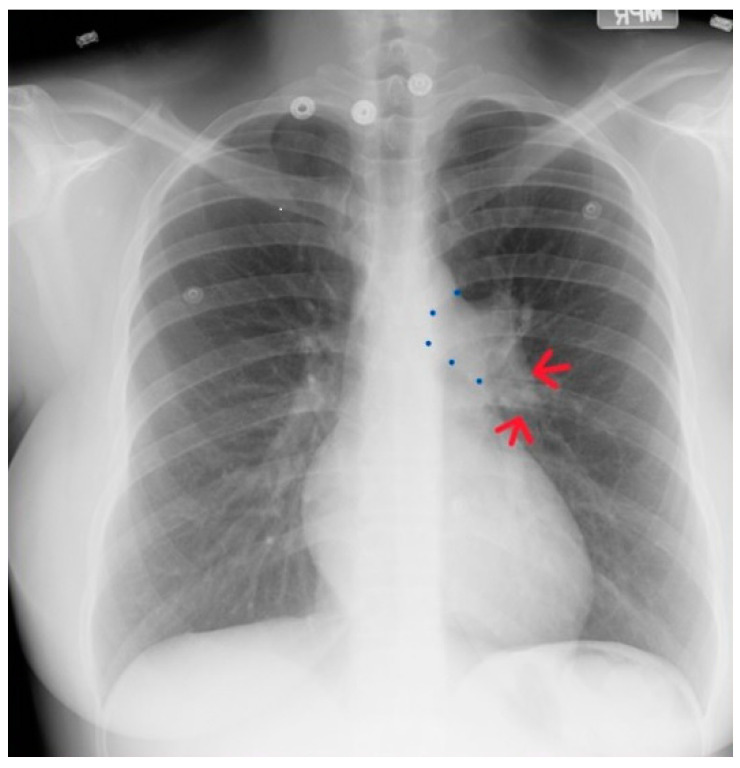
Posteroanterior chest X-ray of severe pulmonary stenosis: this chest X-ray is of a 31-year-old female who presented with worsening exertional dyspnea over the last two years. Her exam revealed elevated jugular venous pressure with prominent A wave. She was noted to have a right ventricular lift with a grade 4/6 systolic ejection murmur best heard at the left upper sternal border. The chest X-ray is well centered and there is a good inspiration. The cardiothoracic ratio is normal. The pulmonary trunk is enlarged (its right border is outlined by blue dots as it passes over the left bronchus). The left pulmonary artery branch that is indicated by two red arrows is also enlarged. Her echocardiogram revealed severe pulmonary valve stenosis with peak gradient 68 mmHg and mild pulmonary valve regurgitation. She subsequently underwent balloon valvuloplasty with reduction of her peak gradient to 24 mmHg. Her symptoms of dyspnea had resolved a few months post-procedure.
